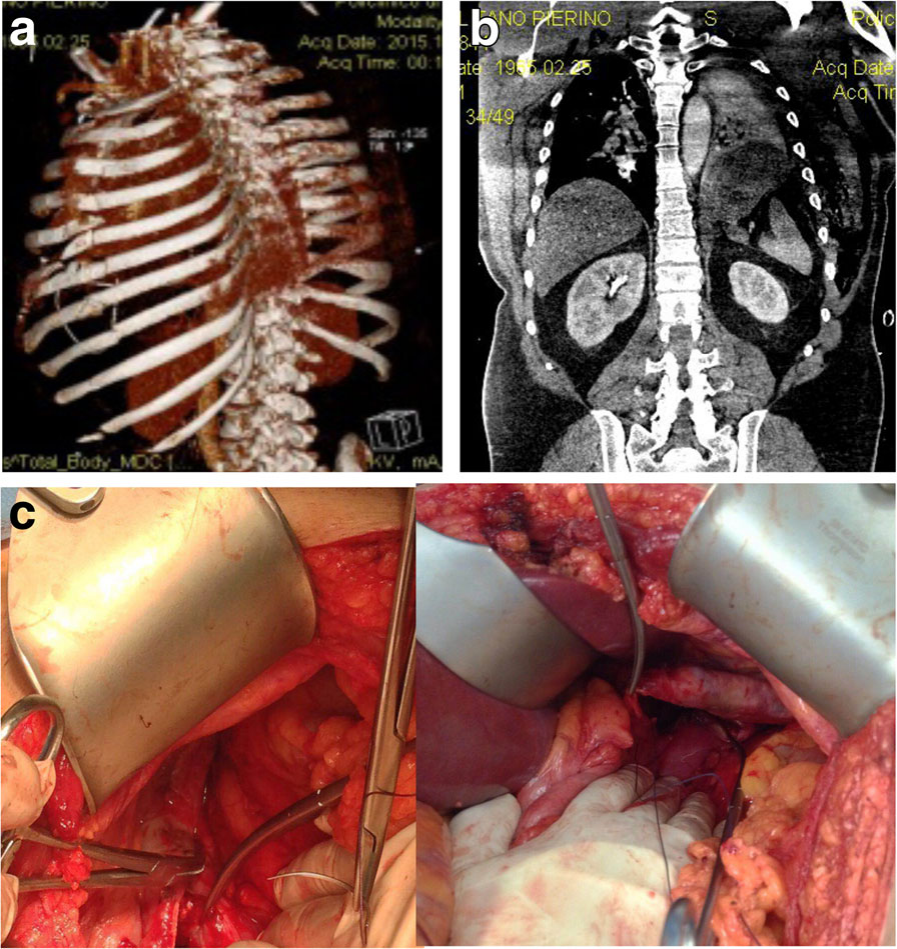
a 3D-CT scan shows rib fractures. b CT scan shows stomach and spleen in the *left* hemi-thorax, complete disruption of all muscular layers. c Intraoperatively, repair of traumatic defect in the *left* diaphragm
